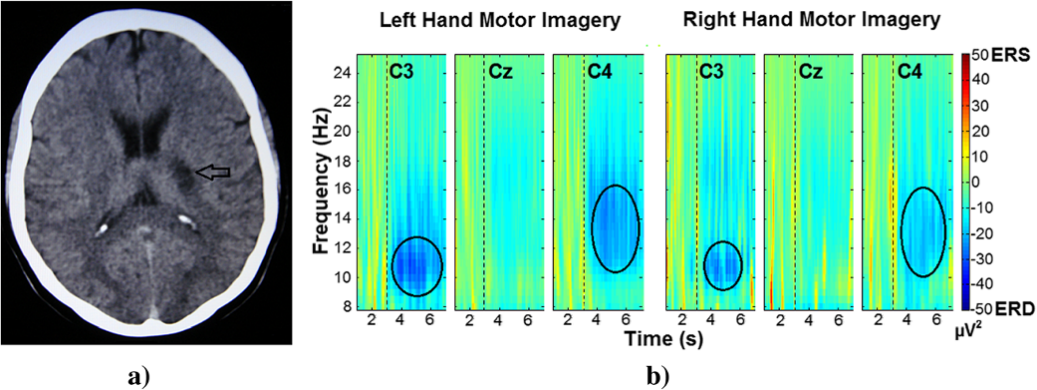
Female patient CT image and averaged TFRs. a) Representative image of the female patient’s CT (Computed Tomography). The black arrow indicates the location of the residual injury. b) Averaged TFR for left and right hand (affected limb) motor imagery in 8 Hz to 25 Hz from 1 s to 7 seconds. Dashed line at 3 seconds indicates the motor imagery onset. The black circles highlight the generated ERD.
